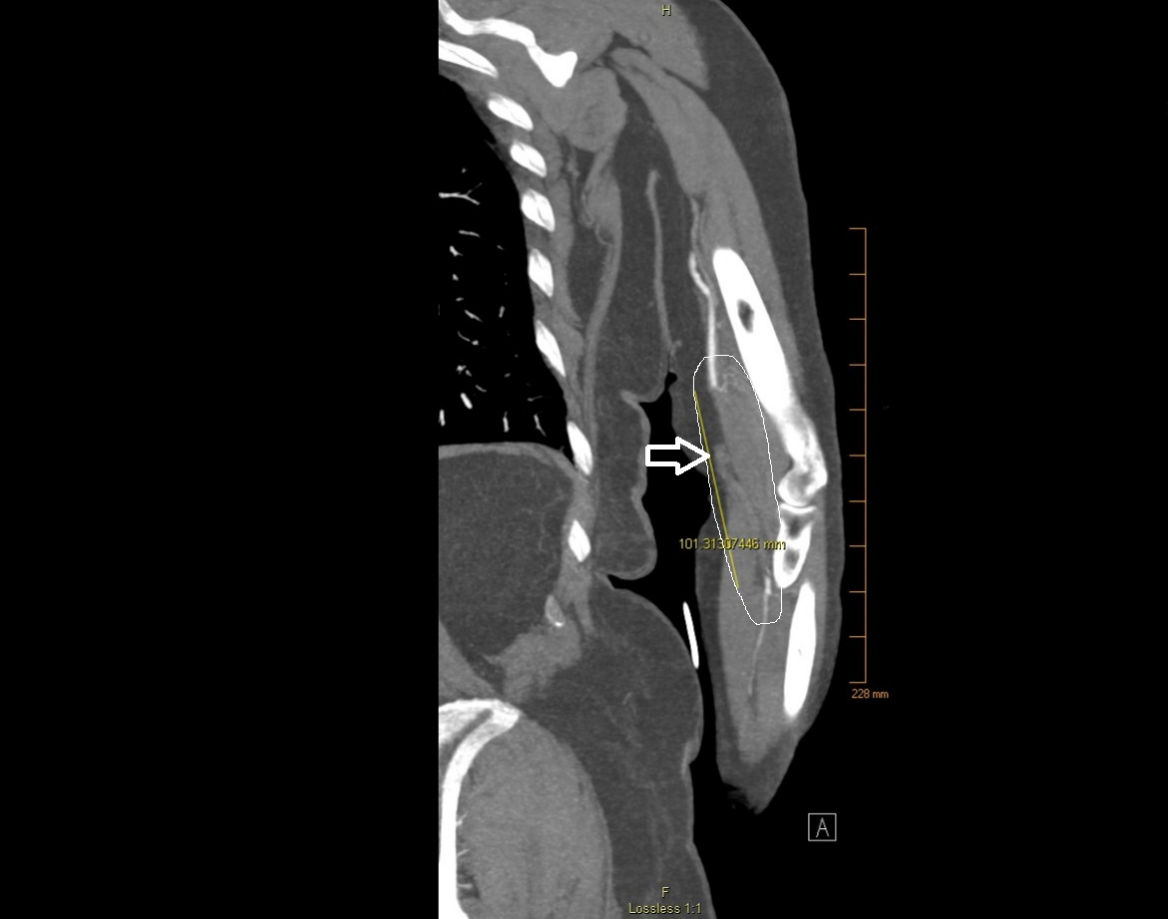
CTA of left upper extremity: Coronal view demonstrating complete occlusion of the distal brachial artery measuring 10 cm in length (outlined and arrow). There is diminished flow in the radial and ulnar arteries. CTA: Computed tomographic angiography
